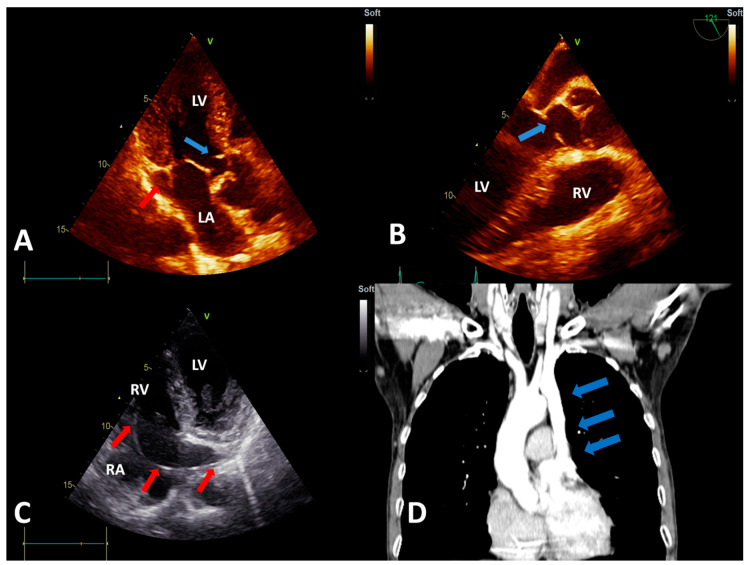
(A) Transthoracic echocardiography (TTE), apical 3-chamber view showing the subaortic membrane (blue arrow) and the dilated coronary sinus (red arrow). (B) Transesophageal echocardiography view showing the subaortic membrane (blue arrow). (C) TTE, modified apical 4-chamber view showing the pacemaker lead (red arrows) entering the right atrium via the dilated coronary sinus (visualized in longitudinal section). (D) Computed tomography angiography showing the persistent left superior vena cava (blue arrows), with an absent right superior vena cava. Abbreviations: LA, left atrium; LV, left ventricle; RA, right atrium; and RV, right ventricle.
